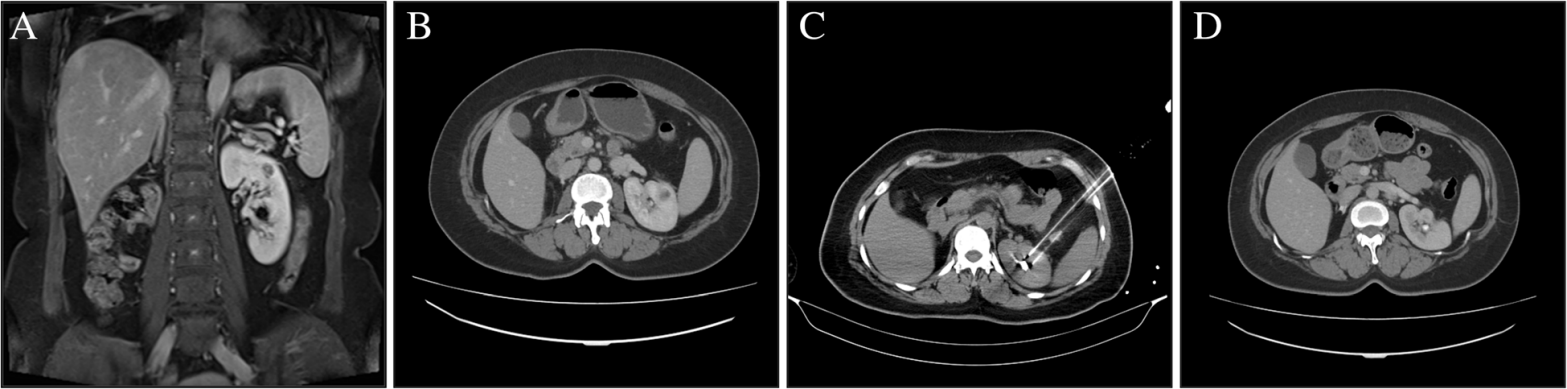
a-b Contrast-enhanced MRI coronal image and contrast-enhanced CT transversal image with the tumor located in the upper pole of the right kidney. c The needles were placed percutaneously through the left colon-spleen space with CT guidance. d Contrast-enhanced CT axial image 15 months after IRE. The kidney parenchyma atrophied, and the tum
